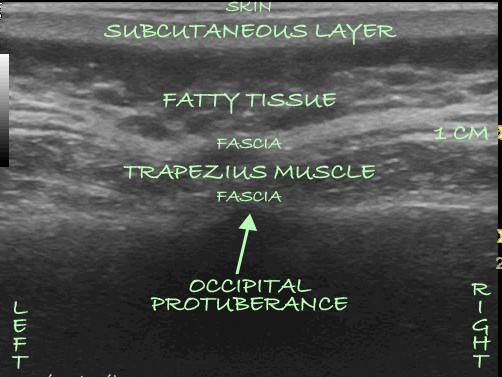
An ultrasound image obtained with a linear transducer placed over the greater occipital protuberance. The anatomical layers are identified sonographically starting from the surface and progressing toward the deeper layers.
